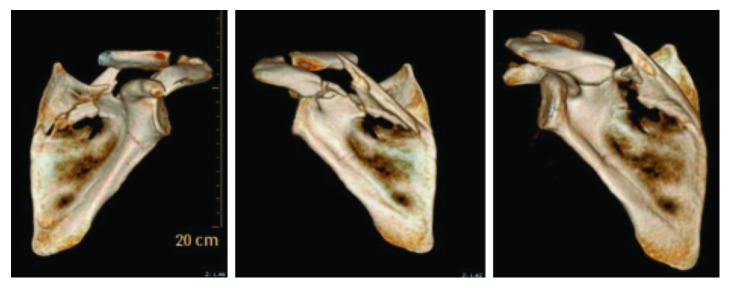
Computed tomography 3-dimensional reconstruction of the scapula showing a segmental fracture of the entire superior aspect including the acromion along with free-floating fragments of its base and spine.
